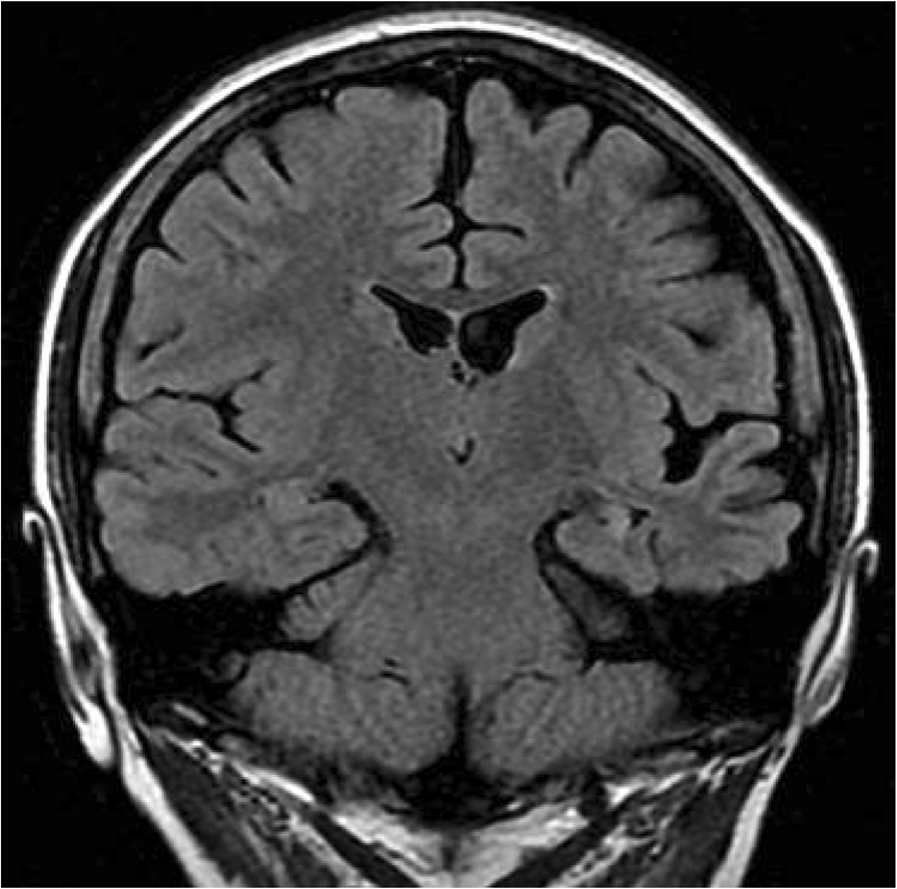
Brain magnetic resonance imaging. Coronal T2-weighted fluid-attenuated inversion recovery image showing left hippocampal hypotrophy, widening of sulci and subarachnoid space in left parietal and temporal lobes, and dilation of the left lateral ventricle
